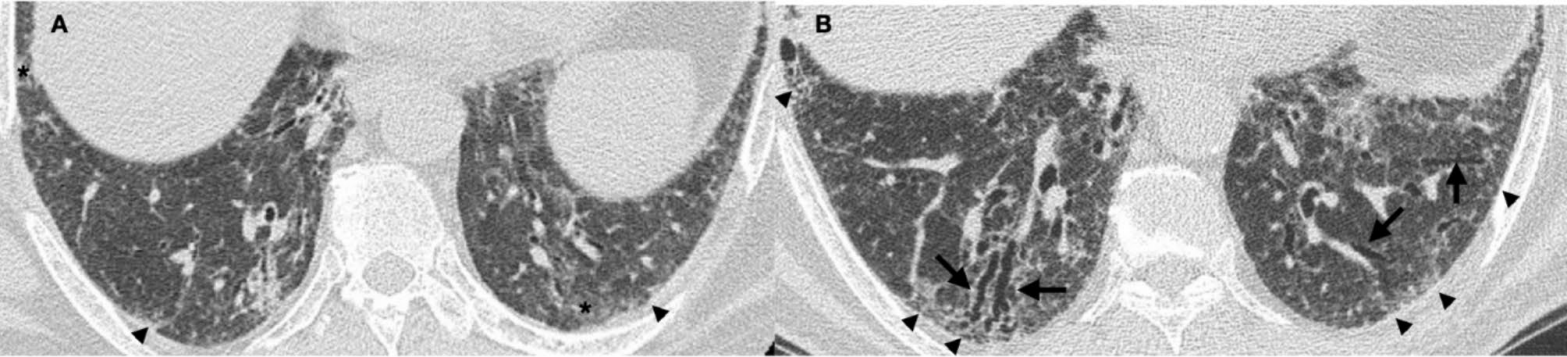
Traction bronchiectasis and disease progression in connective tissue disease-associated interstitial lung disease. A Baseline high-resolution computed tomography (HRCT) shows subtle ground-glass opacities (*) and fine reticulation (arrowheads) with no evidence of bronchiectasis. B Follow-up HRCT after 12 months demonstrates the disease progression with an increase of reticulation (arrowheads) and the appearance of newly developed bronchiectasis (arrows) in both lower lobes
